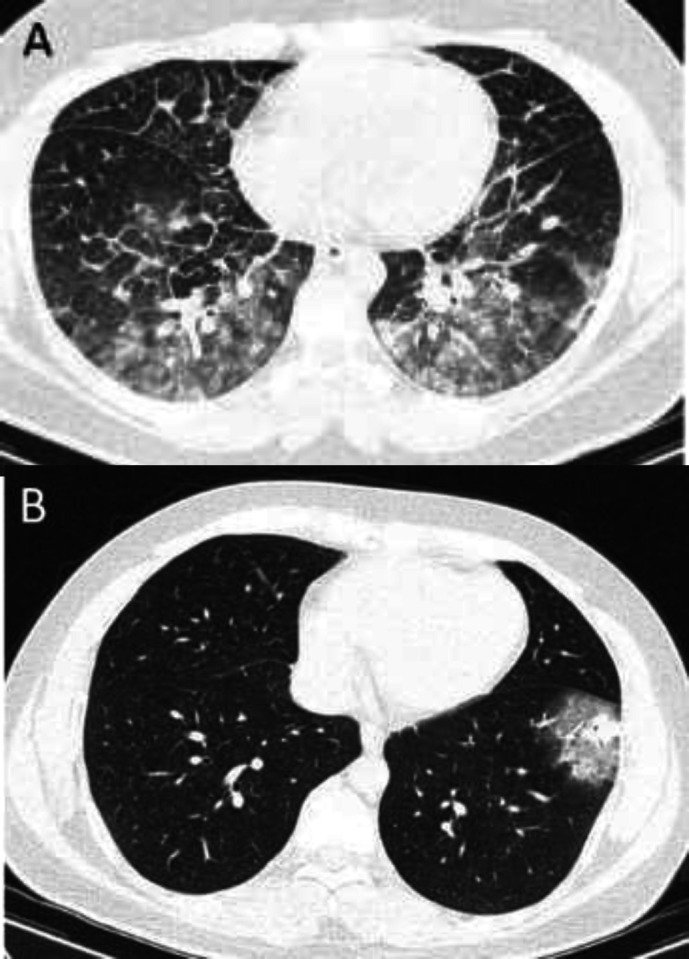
Lung CT scan of both patients. A, lung CT of patient 1 showed bilateral peripheral ground glass opacities in lower lobes, compatible with COVID19. B, lung CT of patient 2 revealed subpleural ground glass density in lower lobe compatible with COVID-19
